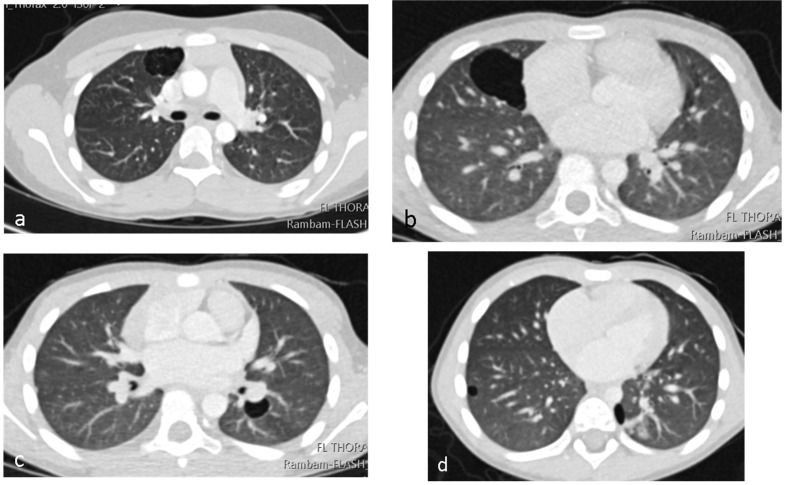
Asymptomatic *DICER1*-germline-positive siblings of index patient number 3. Axial images of Chest CTA: (a) Nine-year-old girl with RUL anterior multiseptated cystic lesion. (b,c) Six-year-old boy with right and left cystic lesion. (d) Fifteen-month-old boy with bilateral, small cystic lesions.
